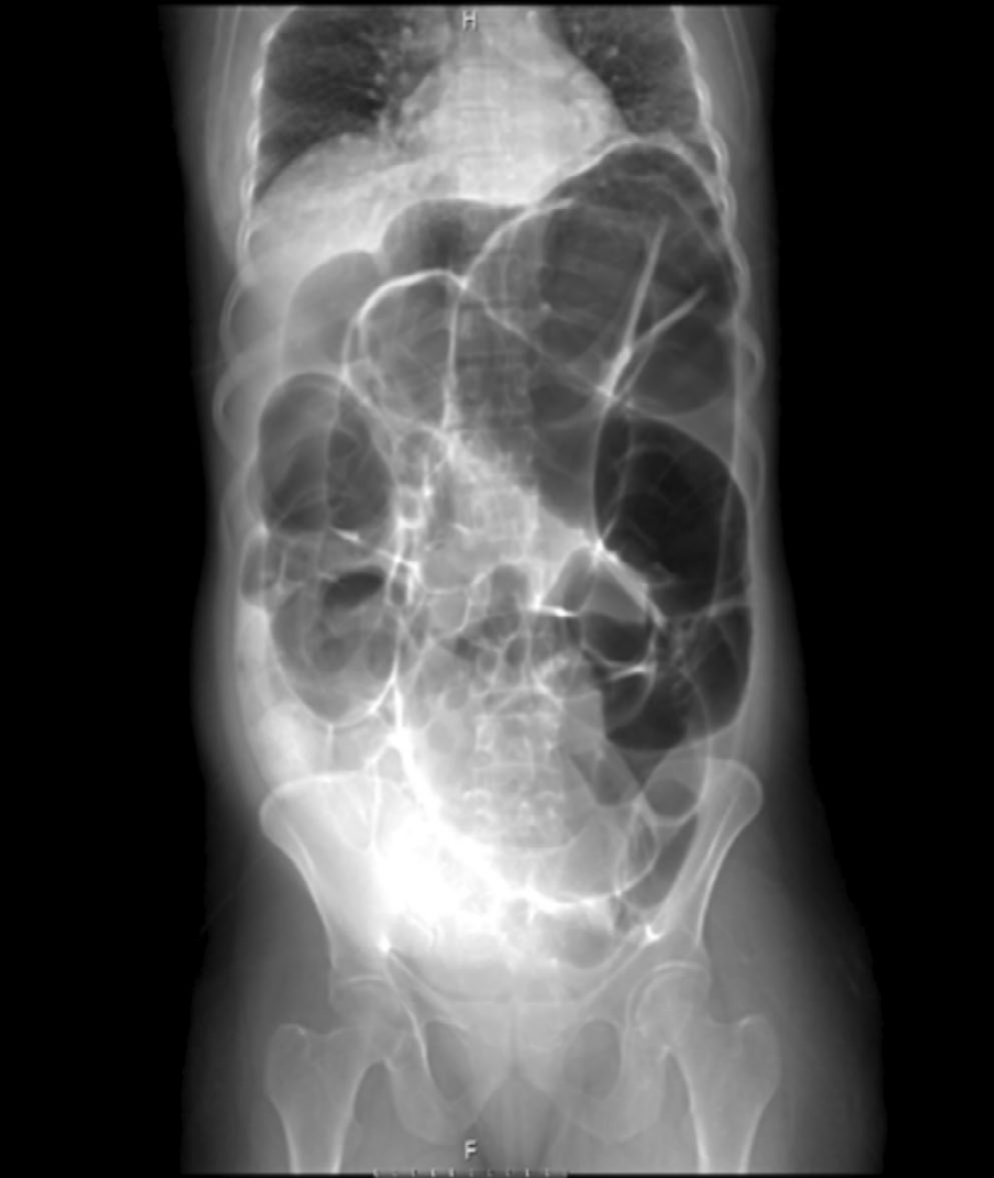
Shows an abdominal radiograph with the classic coffee‐bean sign.
